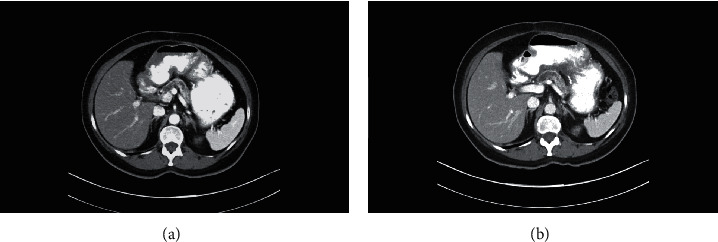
CT scan of abdomen showing a 13.1 × 11.4 × 10.7 cm mass from the posterior aspect of the gastric body (a). Repeat CT scan of abdomen revealing the persistent presence of the mass after chemotherapy with imatinib for 6 months (b).
